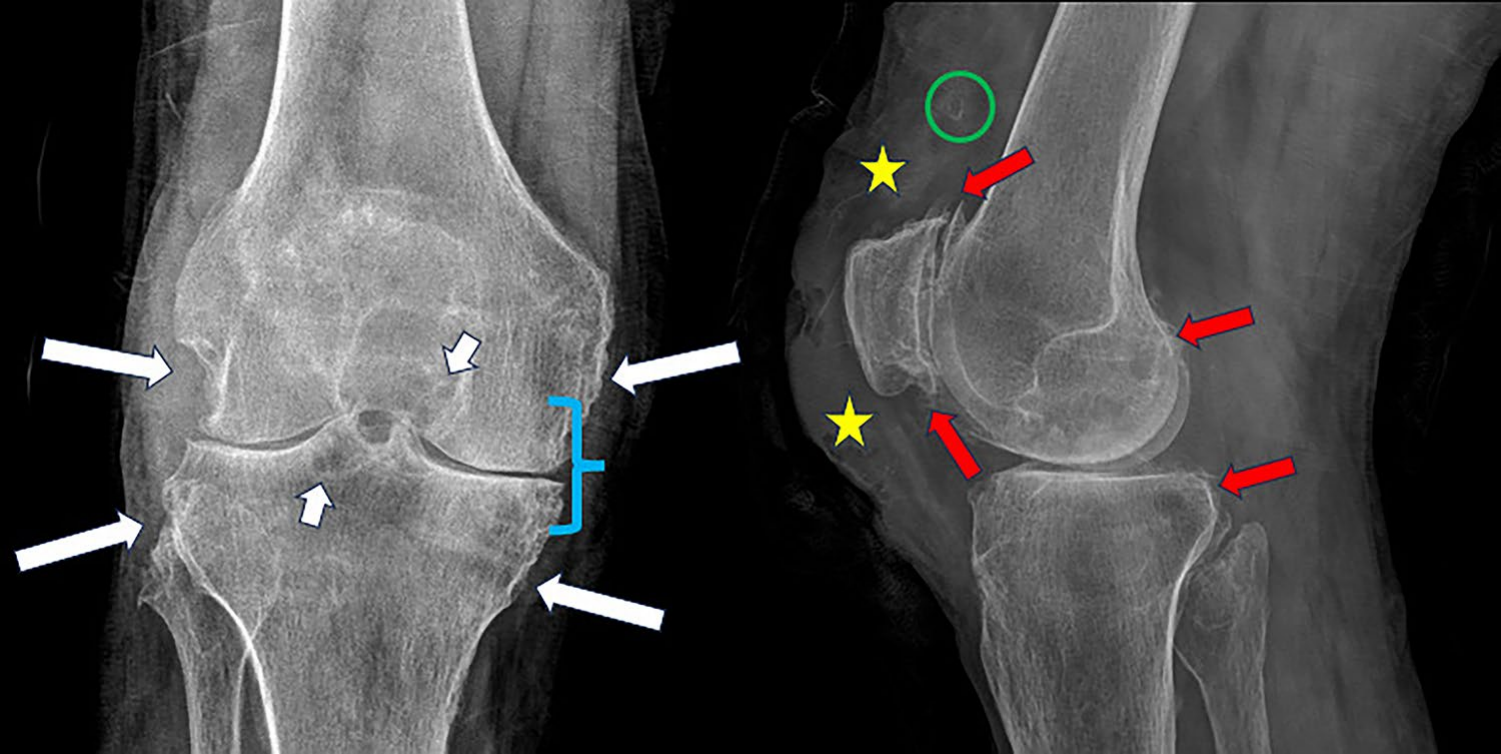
Radiograph of the right knee, anteroposterior (AP) and lateral (L) views, in a 52-year-old man with a 10-year history of chronic tophaceous gout presenting with purulent material oozing from a cutaneous nodule on the right knee. ABCDE’S radiographic approach: A: Adequate alignment. B: Enthesophytes of the extensor mechanism of the patella (red arrows), sclerosis and subchondral cysts (short white arrows) and decreased bone density. C: Symmetrically decreased joint space and increased amount of suprapatellar bursa fluid (blue bracket). D: Single joint. E: Long, parallel, sclerotic, non-marginal femorotibial and peroneal erosions (long white arrows). S: Thickening of the subcutaneous cellular tissue (yellow stars) and calcification projected on the suprapatellar bursa (green circle)
